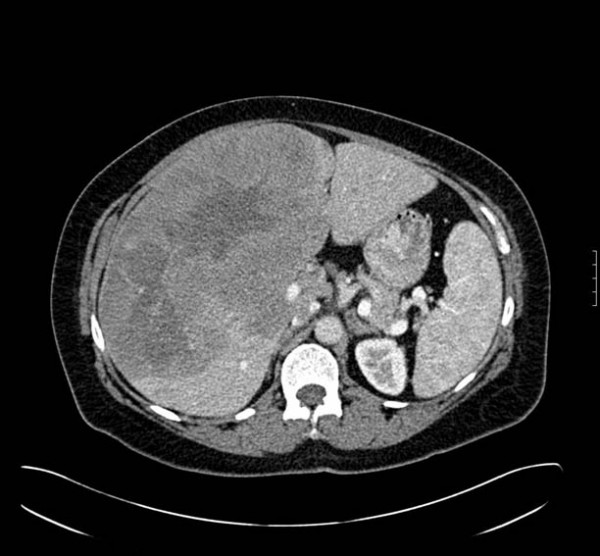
An axial CT image obtained during hepatic arterial phase demonstrates a heterogeneous enhancement of an approximately 22 × 14 cm hepatic mass involving the right hepatic lobe with a central region of hypoattenuation.
